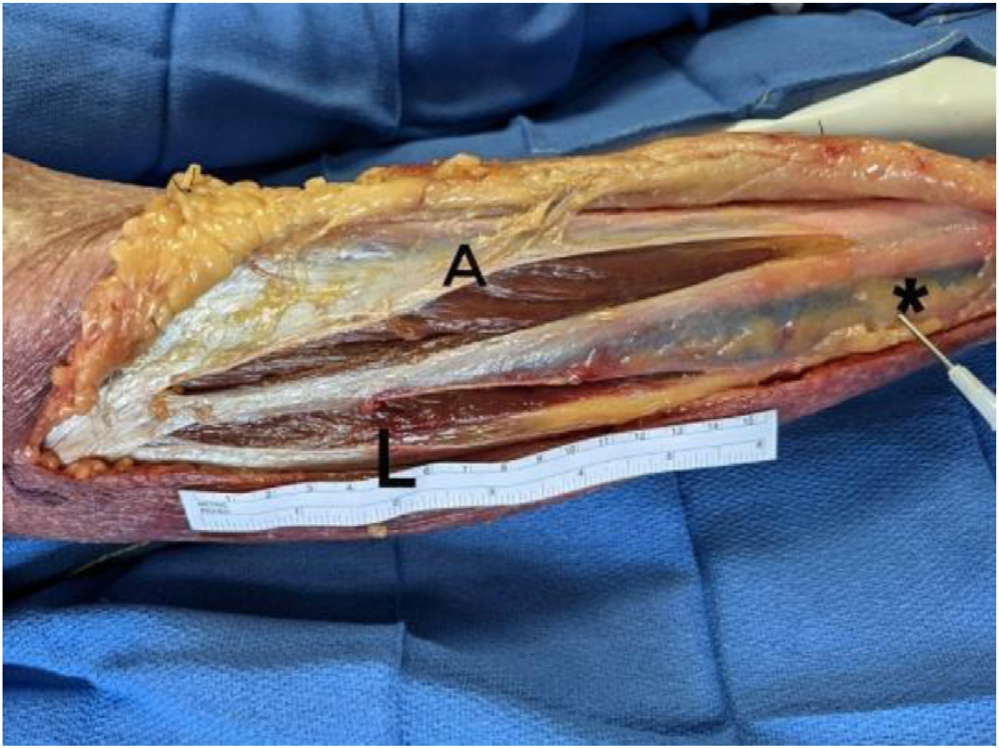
Dissection with measurement of fasciotomy length. The asterisk indicates spotting and marking of the superficial fibular nerve under ultrasound. (A, anterior compartment; L, peroneal compartment.)
